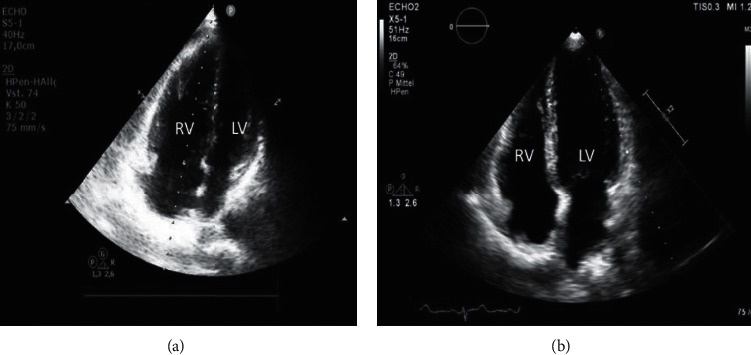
Transthoracic echocardiography, apical four-chamber view, dilated right ventricle RV pre-UACDT (a) and normal size post-UACDT (b) compared to normal-sized left ventricle (LV).
